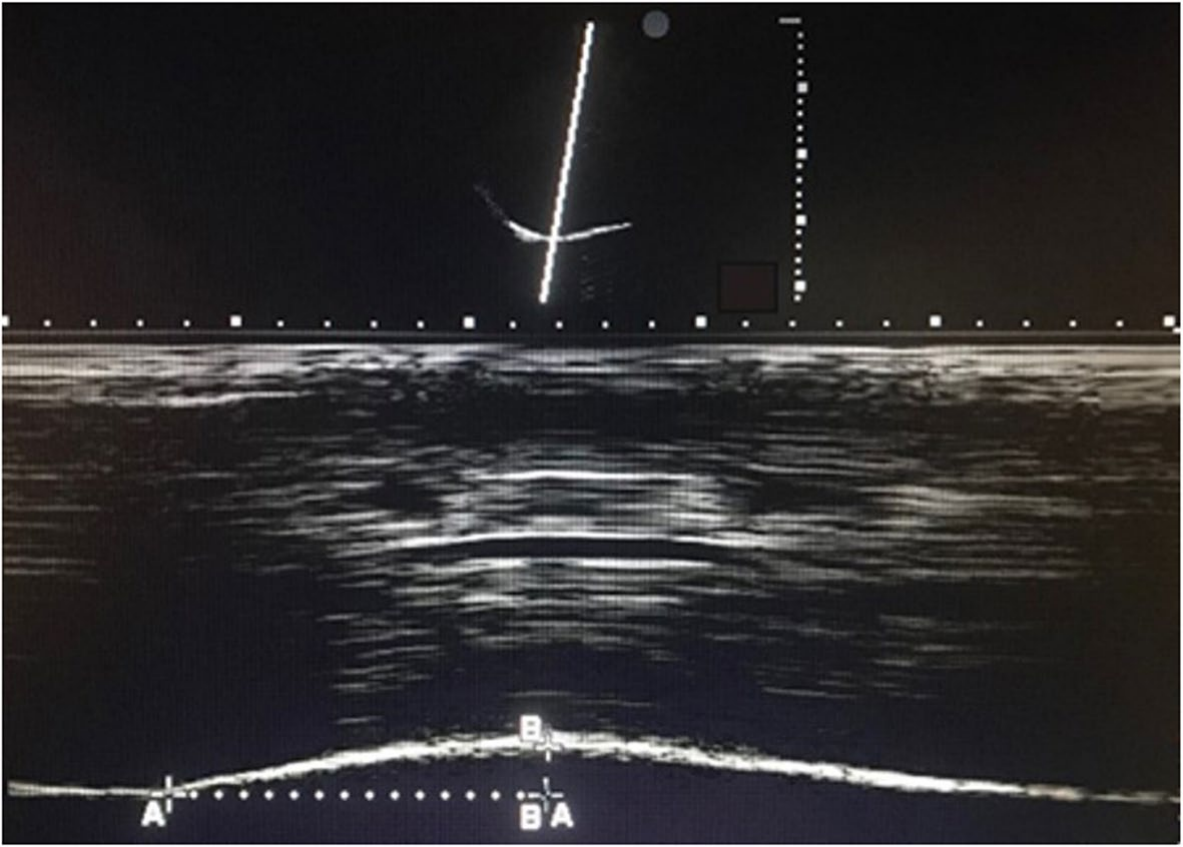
Image demonstrating measurement of the diaphragmatic inspiratory excursion (DE) and time to peak inspiratory amplitude (TPIA) in M-mode ultrasound. A-A is the TPIA. B-B is the DE
